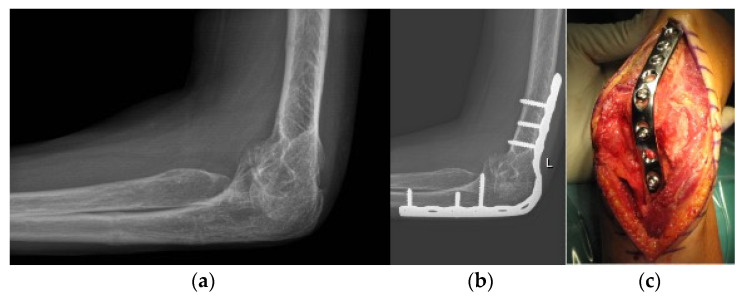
Elbow arthrodesis. (a) preoperative X-ray; (b) postoperative X-ray after elbow arthrodesis; (c) intraoperative image.
